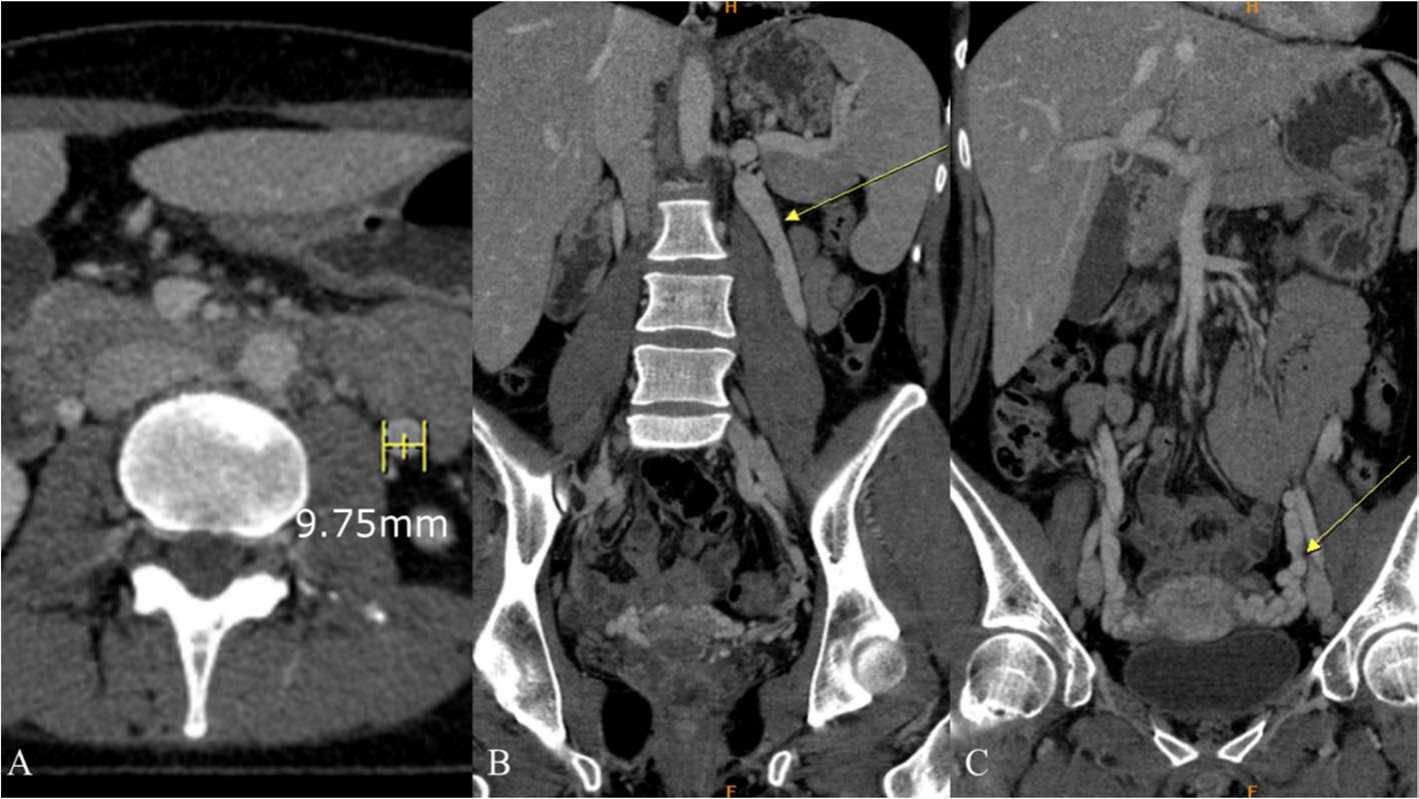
Contrast-enhanced CT examination demonstrating signs of pelvic congestion syndrome distally due to Nutcracker syndrome: A axial plane – dilated left ovarian vein (approximately 10 mm in diameter), B coronal plane – dilated left ovarian vein (arrow), C coronal plane – pelvic varices (arrow)
